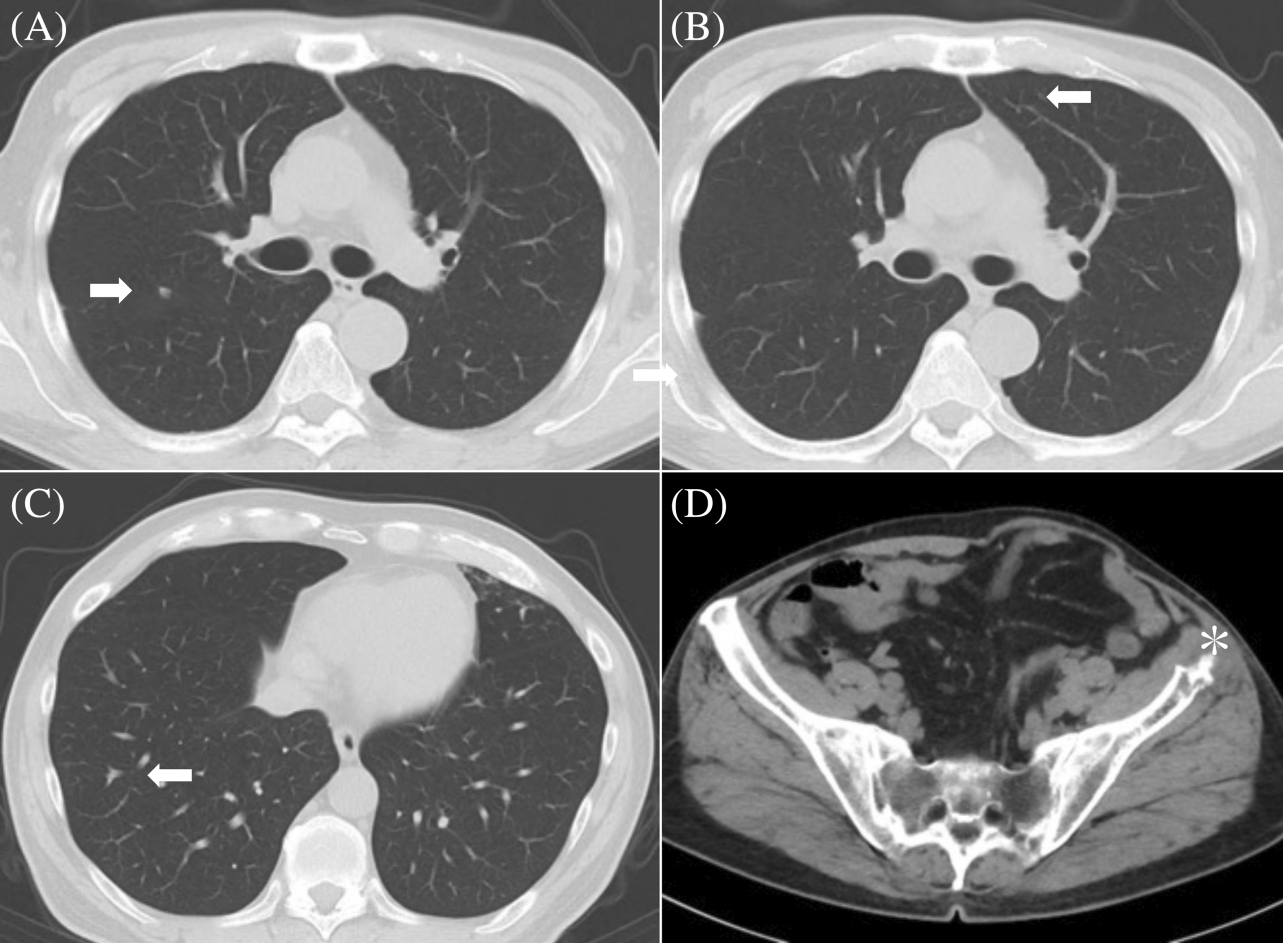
Computed tomography imaging 1 month after radiotherapy. (A, B, C) Multiple lung metastases (white arrows) and (D) iliac bone metastases (asterisks), shown in Figure 2, are observed with remarkable shrinkage.
